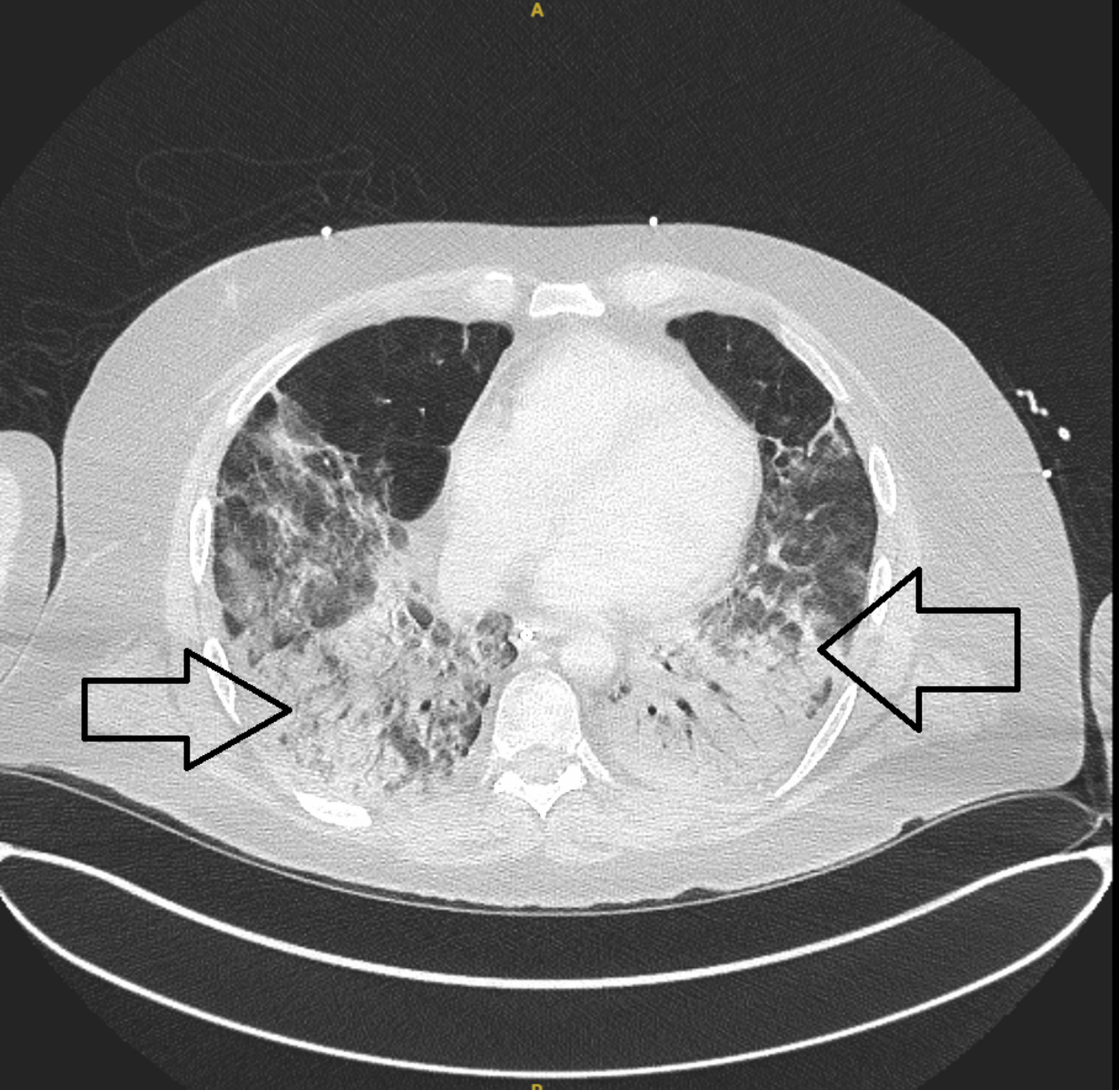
Axial CT chest (August 2022) shows bilateral lower lobe consolidation and ground-glass opacities (black arrows), consistent with organizing pneumonia and active inflammation. This scan corresponds to the initial presentation of hemophagocytic lymphohistiocytosis (HLH), likely infection-triggered. HLH: hemophagocytic lymphohistiocytosis; CT: computed tomography
